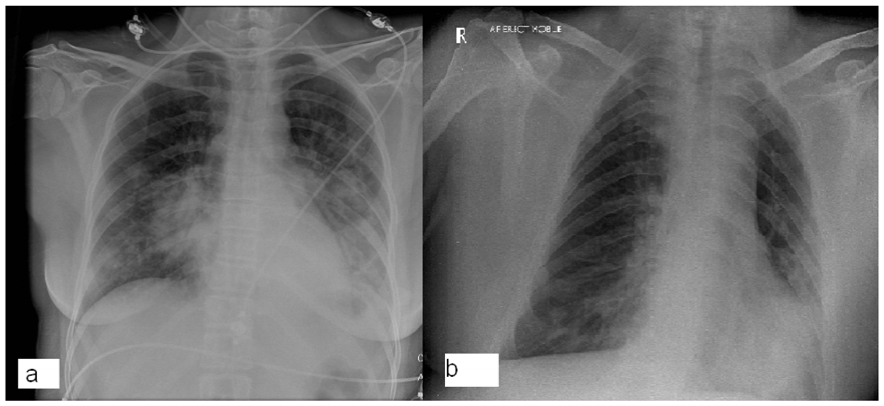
Chest radiography in pandemic A(H1N1)2009/cMRSA co-infection. (a) patient 1, (b) patient 3.
